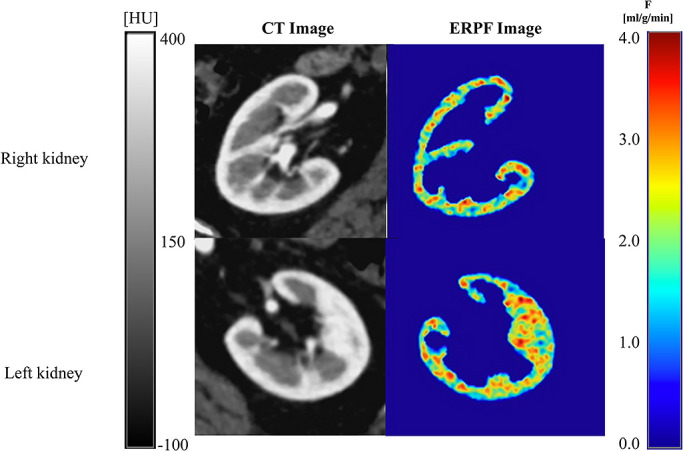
CT image and CT-ERPF image. Left: Contrast-enhanced CT images. Right: CT-ERPF images. The
